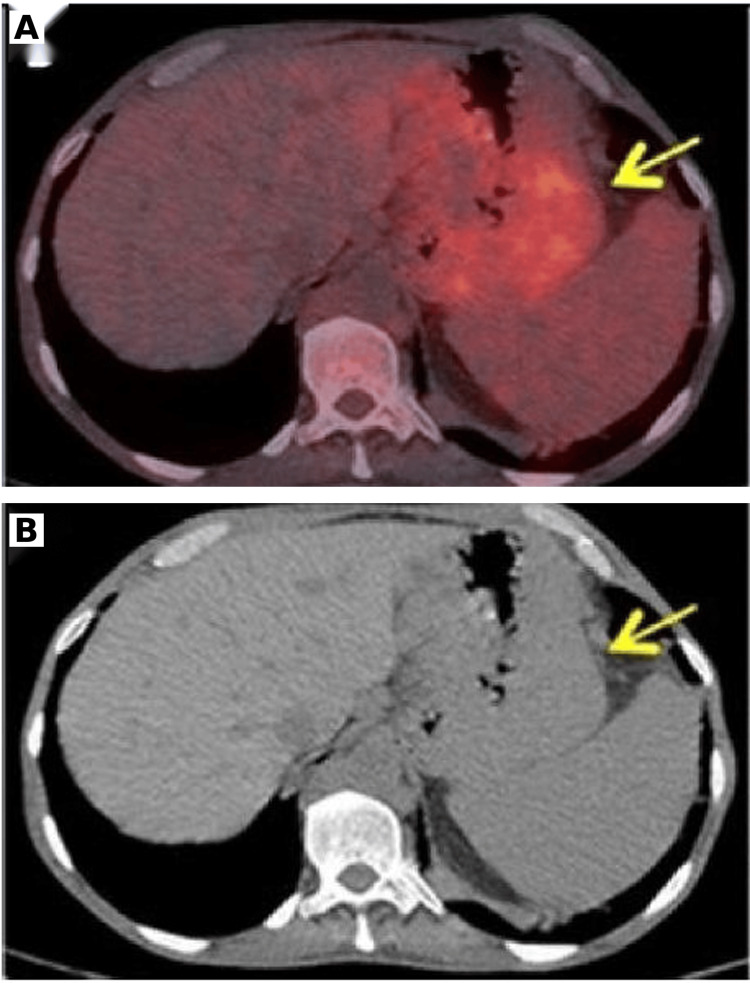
Cross-sectional imaging of the abdomen demonstrating hepatosplenomegaly. (A) Axial PET/CT fusion image showing focal FDG-avid uptake in the spleen (yellow arrow), with diffusely enlarged liver and spleen. (B) Corresponding axial non-contrast CT image at the same level demonstrating splenomegaly with a hypodense splenic lesion consistent with infarction (yellow arrow). No biliary ductal dilation was identified
